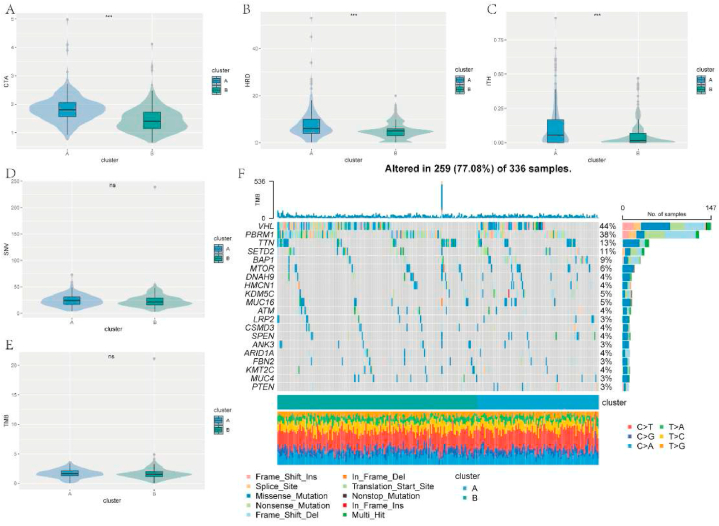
Analysis of the immunogenicity-related features in the molecular clusters. A-C. Different levels of CTA, HRD, and ITH among cluster A and B. D-E. No significant difference of SNV and TMB scores were recognized between the molecular clusters. F. A waterfall plot displaying the top 20 altered genes in cluster A and B.
